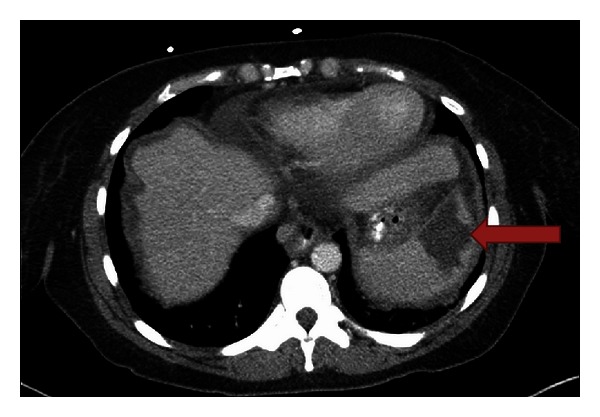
Axial CT scan showing a wedge shaped low-attenuation area in the spleen representing an area of infarction (red arrow).
